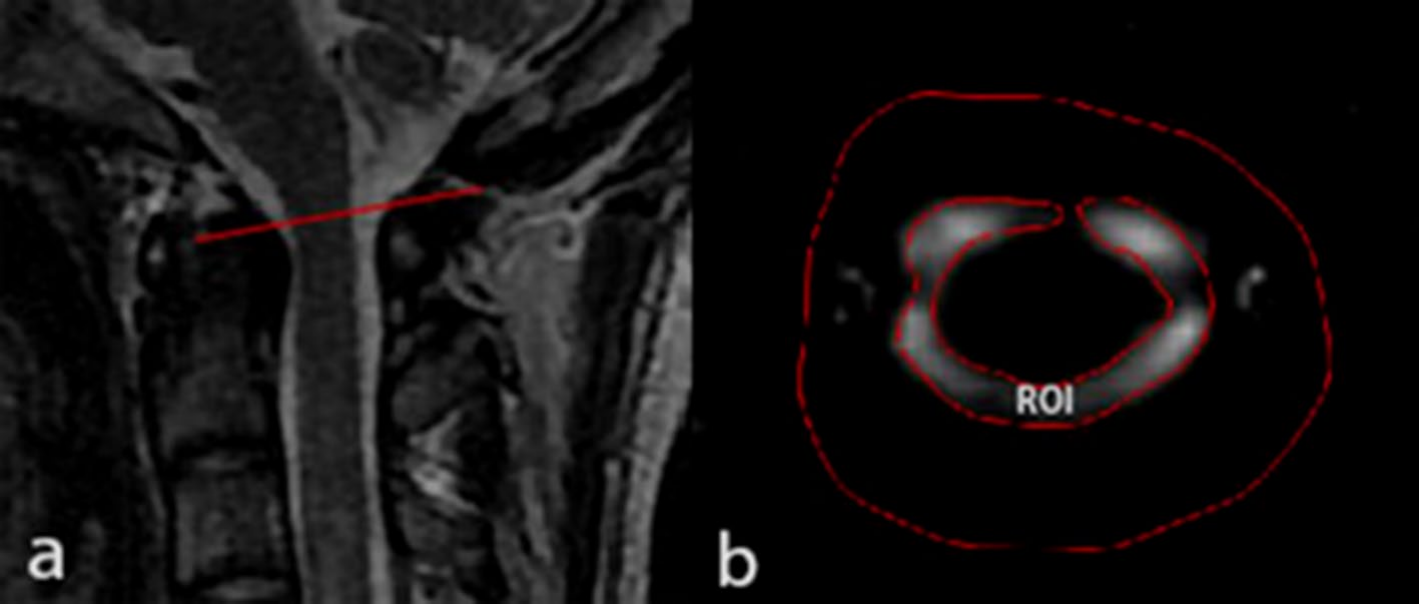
Example of MR images from one of the volunteers. The region of interest is the entire cross-sectional area of the subarachnoid space at the level of the upper border of atlas. (a) Showing the level of upper border of the atlas (red line) in the median sagittal plane of nape; (b) the region of interesting (ROI, inner closed irregular contour) showing the entire cross-sectional area of the subarachnoid space in the transverse plane at the level of the upper border of atlas. And the outer red line serves as a guideline.
